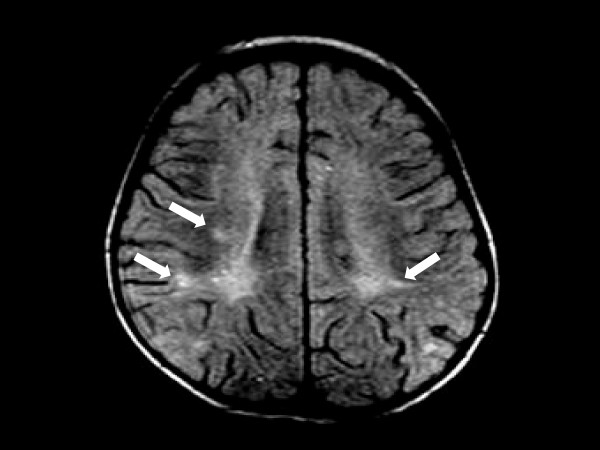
MRI scan showing ill-defined hyperintense lesions at the peri-ventricular and subcortical white matter (arrows) that were identified shortly after the skin eruption and the *Streptococcus pneumoniae *growth.
